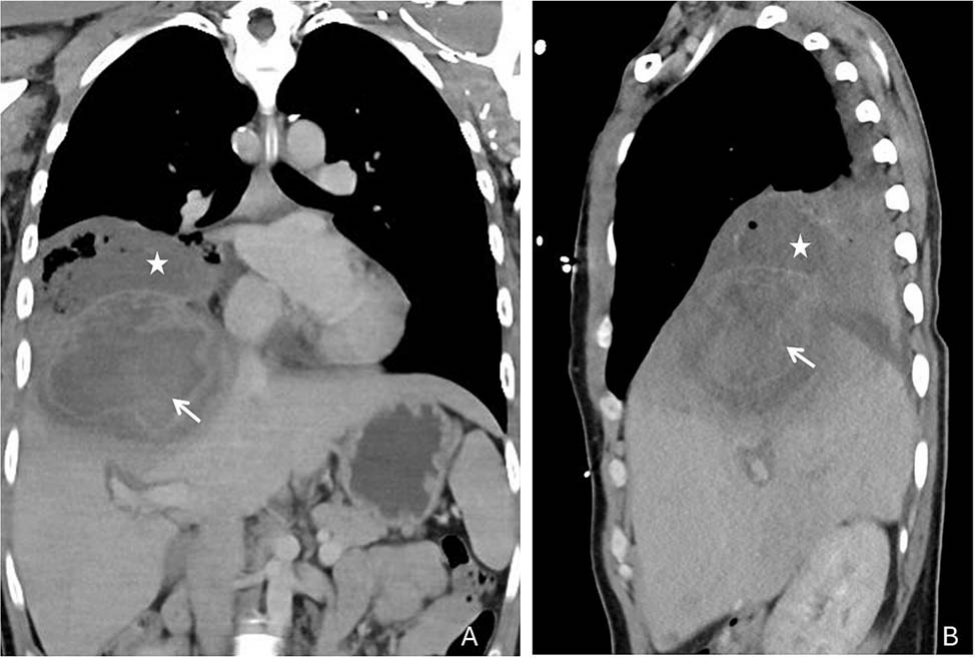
Coronal (A) and sagittal (B) reconstructions of the thoracoabdominal computed tomography revealed a peripherally contrast-enhancing collection with perifocal edema in liver segments VII/VIII (white arrows) communicating with the right broncho-pleural space (white stars)
